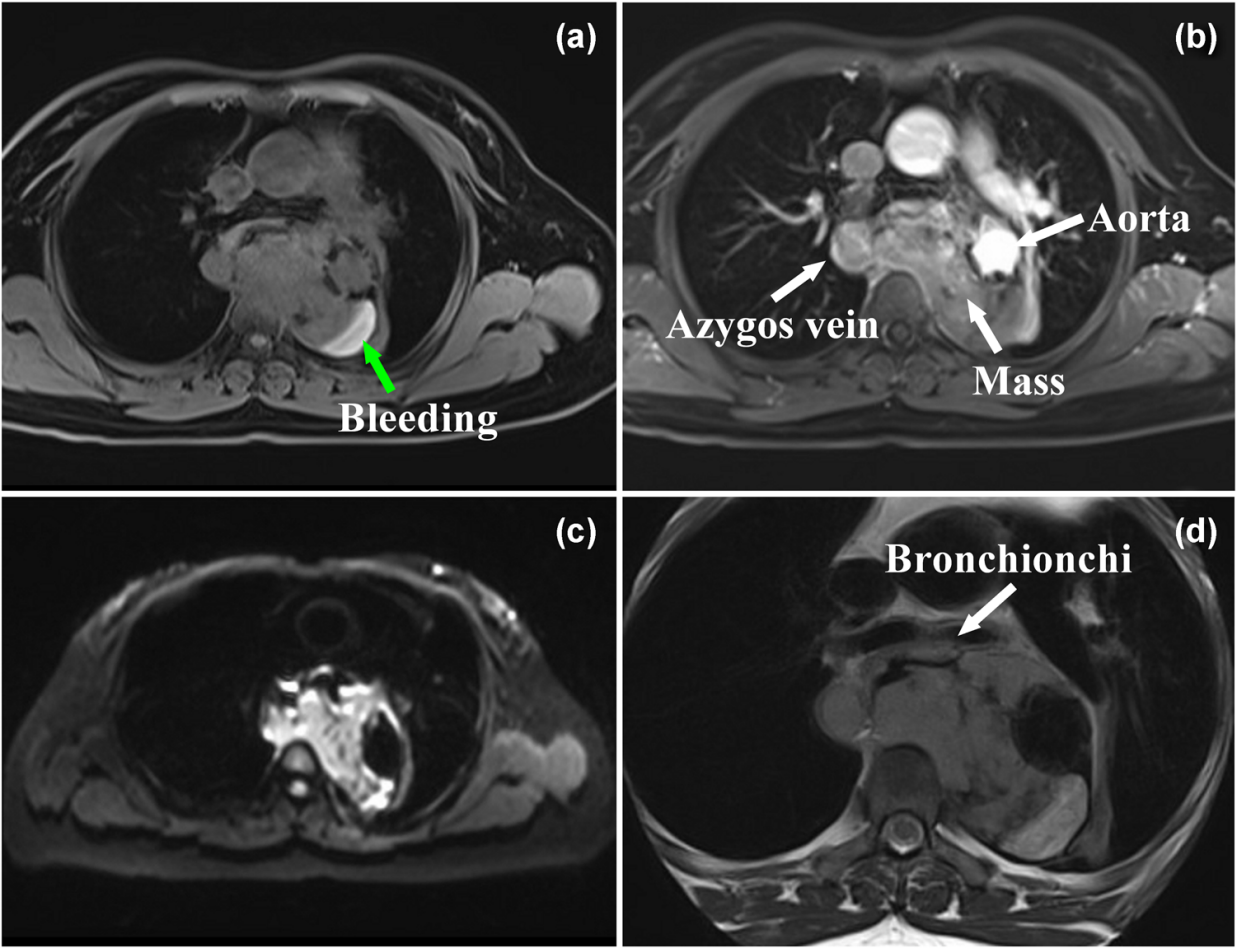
Magnetic resonance imaging showing gradual enlargement of false lumen with mediastinal extensions and a new lesion in the azygos vein. T_1_WI image (a) and T_2_WI image (d) showing the heterogeneous of “false lumen” with bleeding area (green arrow). Contrast-enhanced T_1_WI image (b) showing a nonhomogeneous enhancement of the “false lumen,” which correspond to the hyperintense regions in DWI (*b* = 800 s/mm^2^) image (c).
